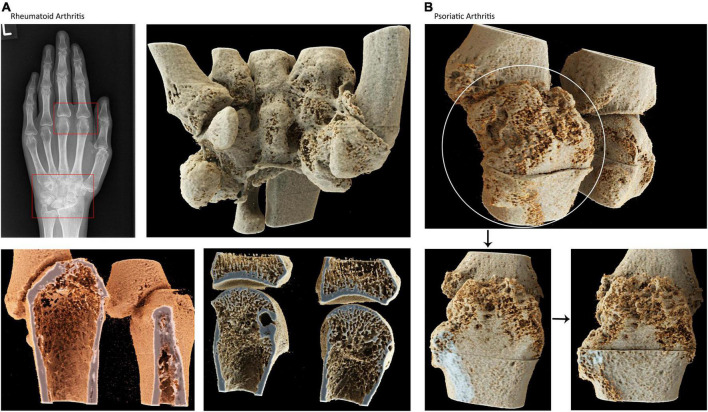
Cinematic rendering of the hand joints in rheumatoid and psoriatic arthritis. (A) Conventional radiographs of the hand of a patient with rheumatoid arthritis showing carpal and metacarophalangeal pathologies. Cinematic rendering based on high-resolution CT images showing (B) carpal bones with deformities and erosions (upper row) peripheral, trabecular demineralization and erosive changes in RA. This technique enables photorealistic depiction of the inner life of the human bone (B) cinematic rendering based on high-resolution CT images showing bone erosion and new bone formation in psoriatic arthritis self-explaining the difference between RA and PsA.
